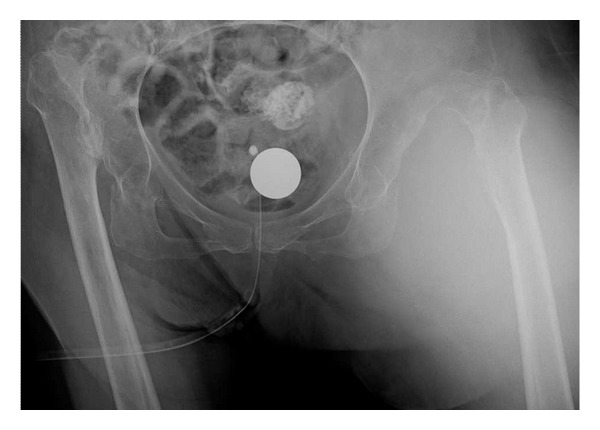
Radiograph taken at admission.
